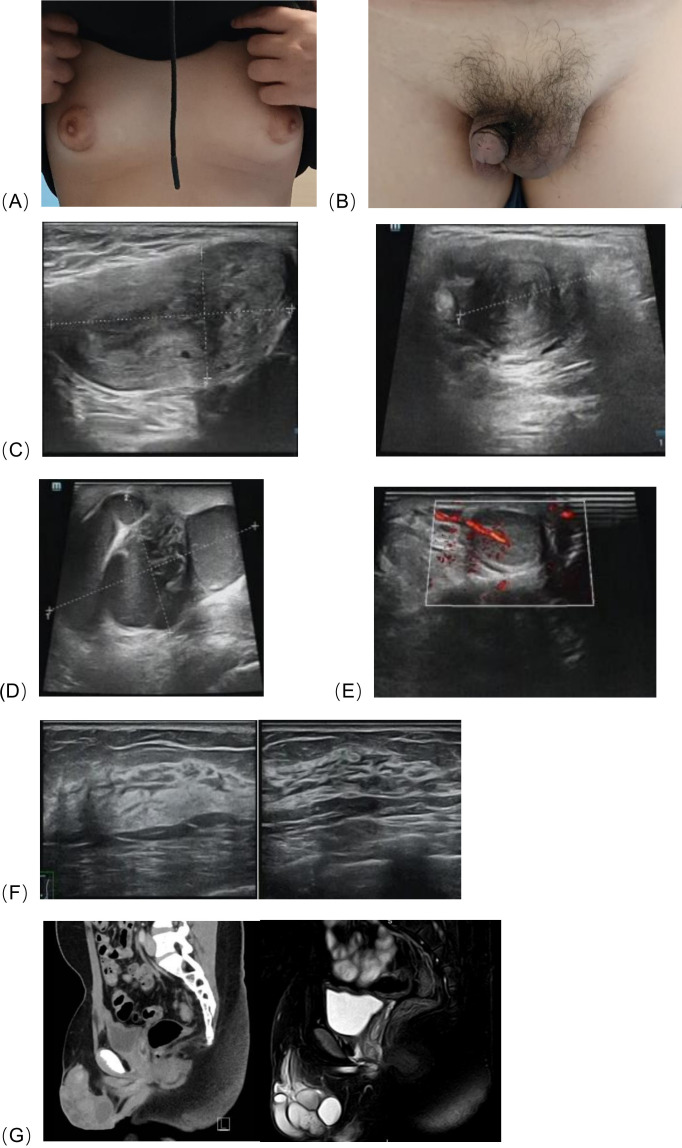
(A) Bilateral breast development, (B) The patient’s external genitalia, (C) Ultrasonography revealed an abnormal solid mass in the left inguinal region and scrotum, consistent with a uterus, measuring approximately 5.7×3.0×2.4 cm, with endometrial echo (thickness ~0.7 cm) and uterine cavity separation (~0.4 cm). (D)Surrounding the mass were tortuous, dilated tubular anechoic areas, suggestive of fallopian tubes, measuring approximately 7.1×4.2×6.1 cm. (E) The right scrotum contained testicular parenchyma, measuring 2.0×1.4×0.8 cm. (F) Breast ultrasound showed glandular tissue in both breasts, measuring 1.2 cm in anteroposterior diameter, with no detectable masses. (G) CT and MRI revealed a tubular soft tissue structure with a lumen, measuring approximately 4.8×1.5×1.1 cm, was detected between the bladder and rectum.
